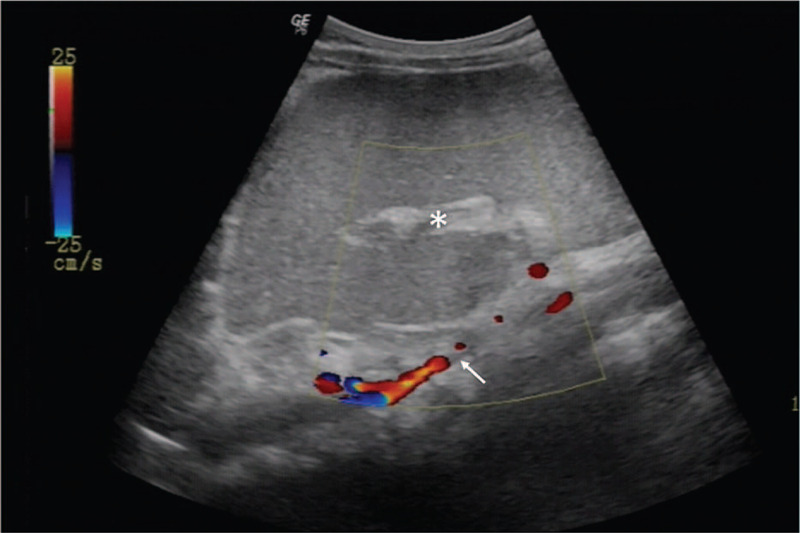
Ultrasound of the abdominal mass. The heterogeneous spleen presents a hyperechoic texture (marked in ^∗^) with absent intraparenchymal color Doppler flow from the twisting point on the vascular pedicle (arrow).
